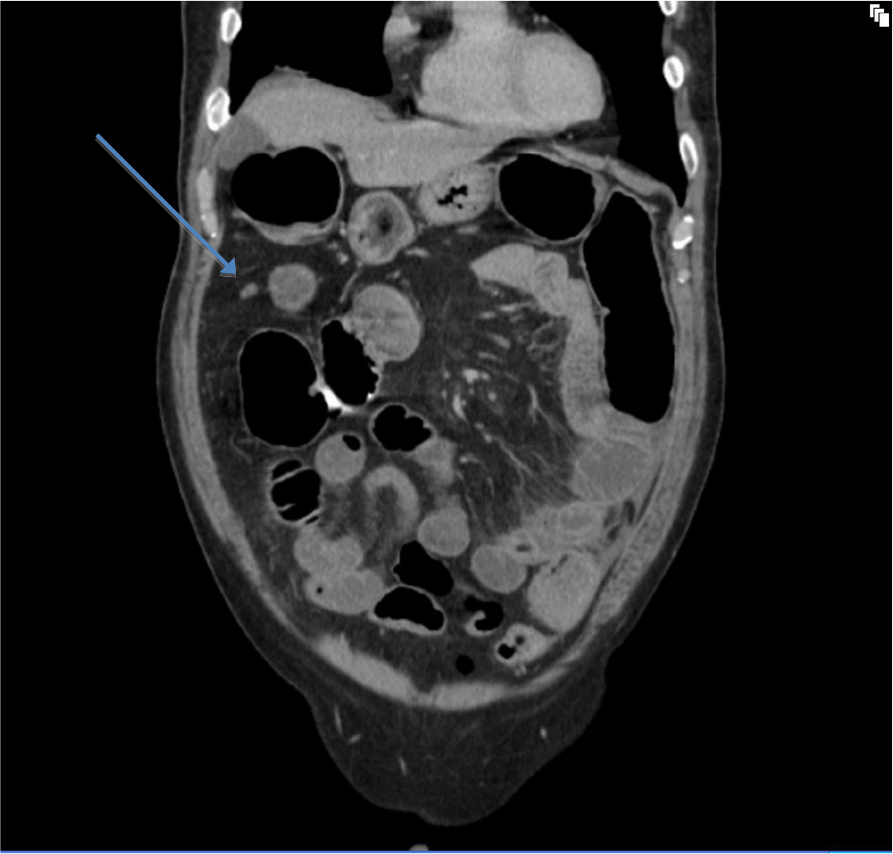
Coronal section of the CT scan. The blue arrow is pointing to a small round lesion in the right upper quadrant, most likely representing the omental lesion.
